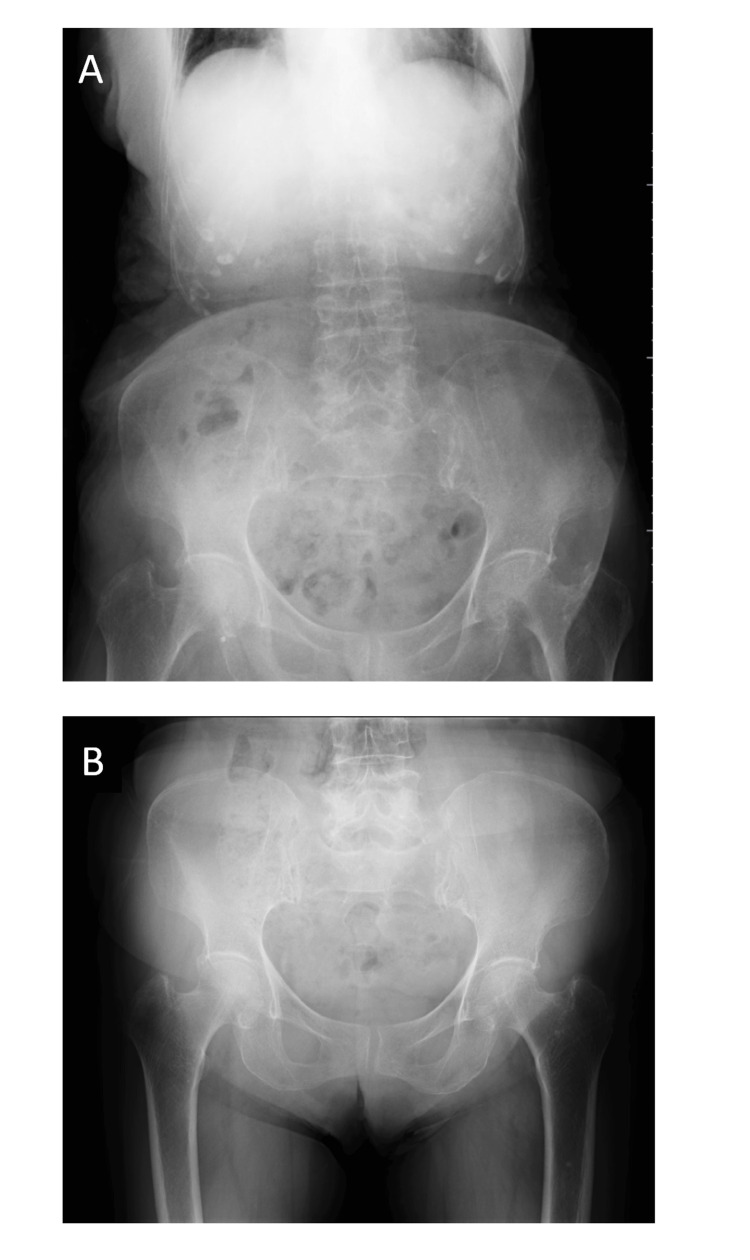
Abdominal roentgenogram and pelvic radiograph before admission (A) An abdominal roentgenogram in the supine position showing no dilated small intestinal loops and no abnormal intestinal gas. The degree of intestinal dilatation was slight because she had just developed ileus a few hours earlier. (B) The pelvic radiograph showing no abnormalities of the bones or joints in spite of her right thigh pain.
